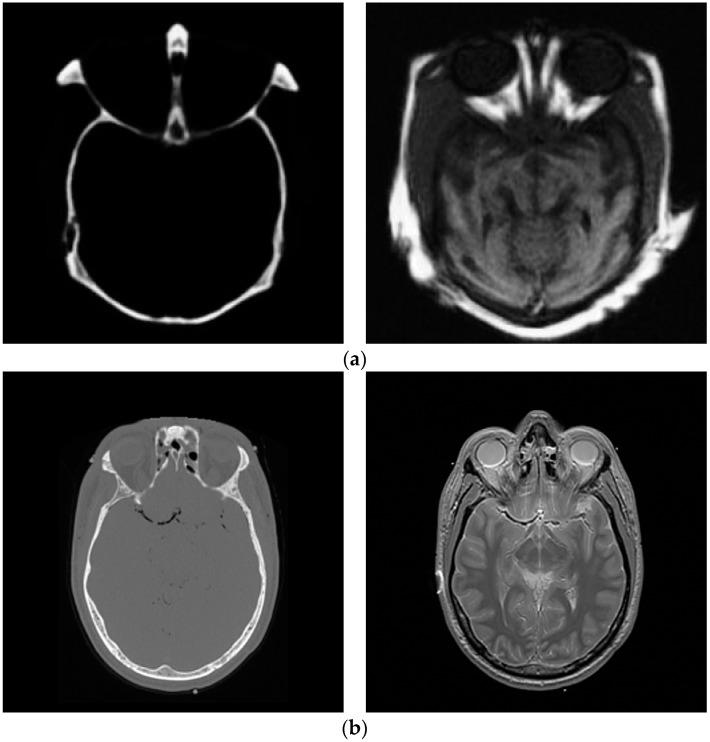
Multi-modal images, (a) CT and (b) MRI images.
